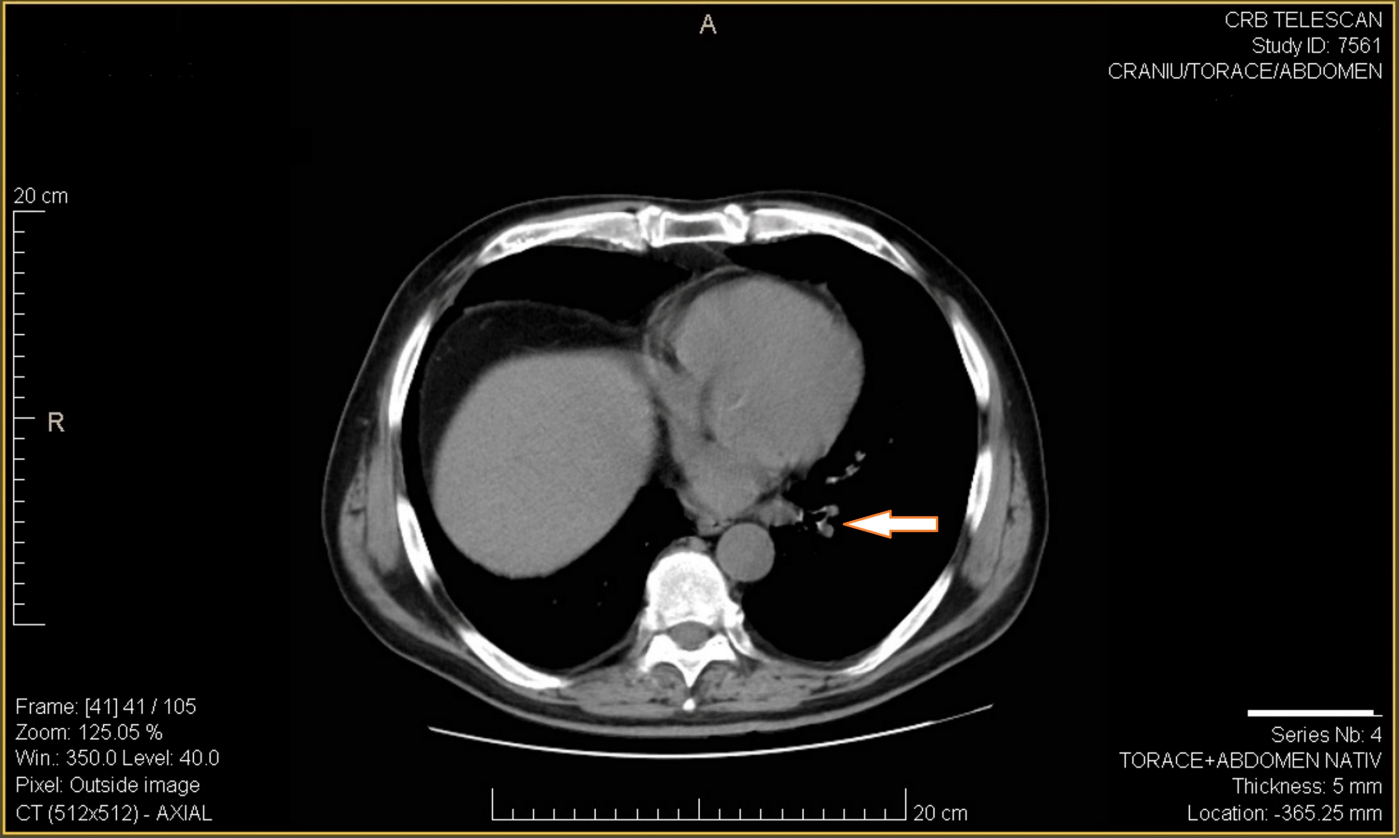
CT scan showing the "de novo" appearance of two left nodular lesions
